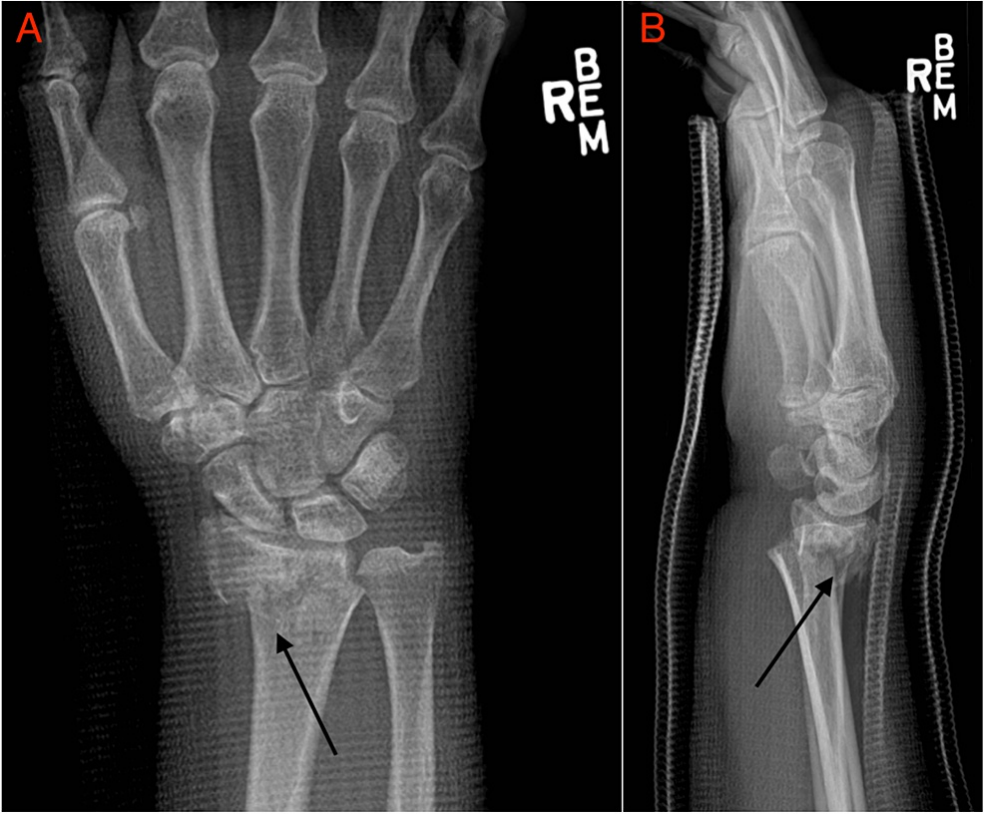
Posteroanterior (A) and lateral (B) radiographs of the right wrist were performed in the emergency department prior to closed reduction. There is an extra-articular distal radius fracture with dorsal and radial translation of the distal fragment and approximately 20 degrees of dorsal angulation.
